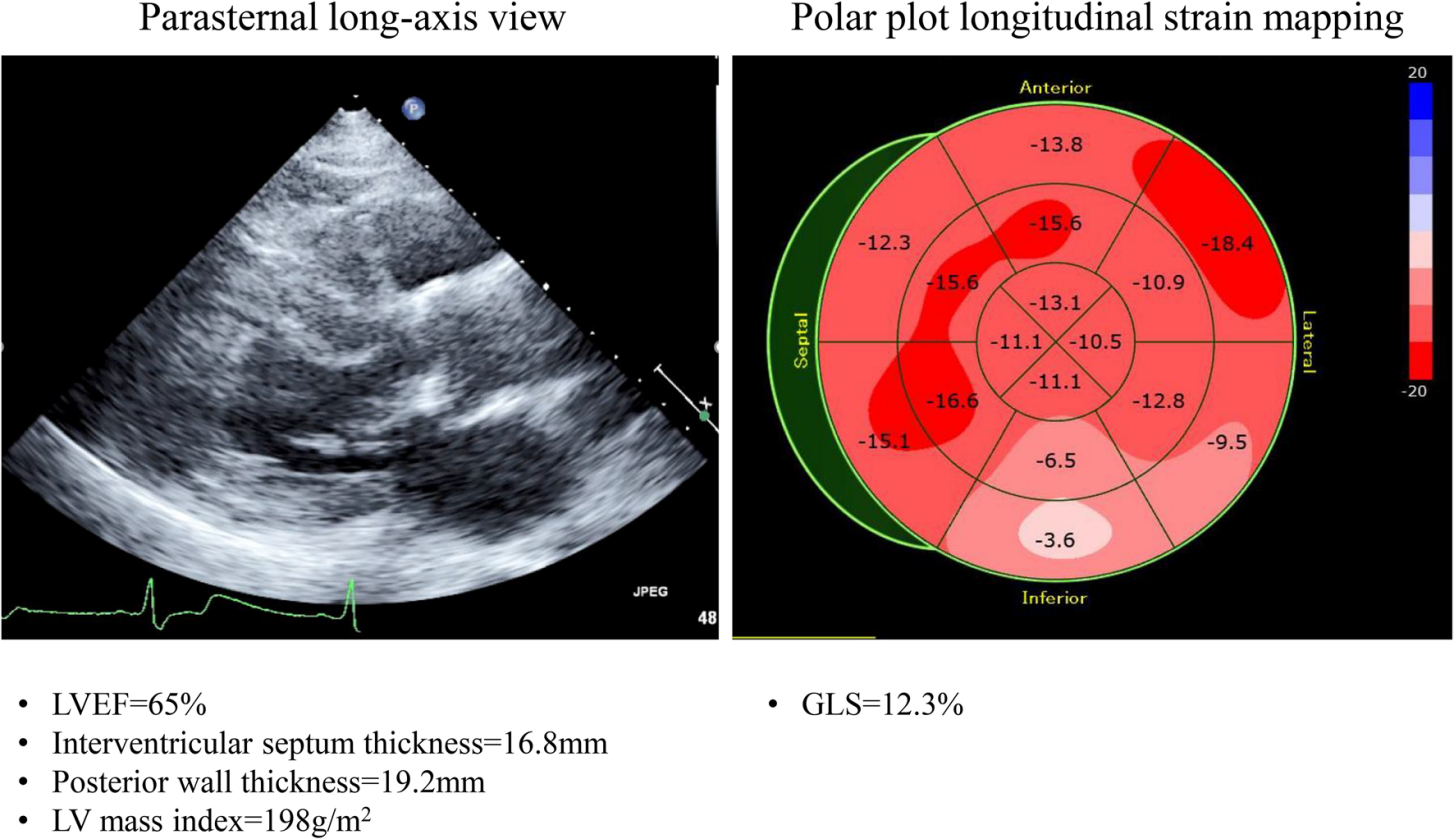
Examples of the parasternal long-axis view and the longitudinal strain bull’s eye plot for a 72-year-old female with Fabry disease. LVEF of 65% is preserved without wall motion abnormality, but GLS of 12.3% is low. Furthermore, longitudinal strain in the basal posterior-lateral wall was further reduced compared to that in other regions
